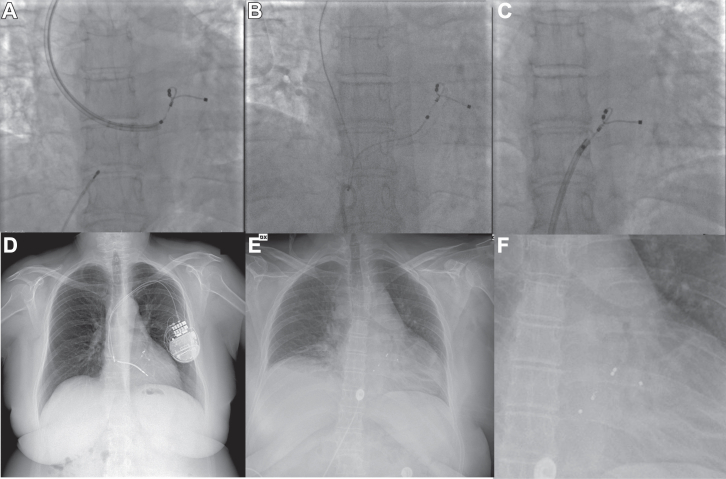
Attain Stability lead (Medtronic, Minneapolis, MN) extraction procedure. A: Mechanical dilation (MD) of adherence at the coronary sinus (CS) ostium through subclavian venous approach with polypropylene sheath. B: Externalization of the Attain Stability from the venous entry site to the right venous femoral access. C: MD of adherence at the CS ostium through right venous femoral access with the Agilis introducer (Abbott, Chicago, IL). D: Chest radiography before extraction. E: Chest radiography after extraction. F: Chest radiography after extraction with focus on the abandoned tip of the CS lead.
